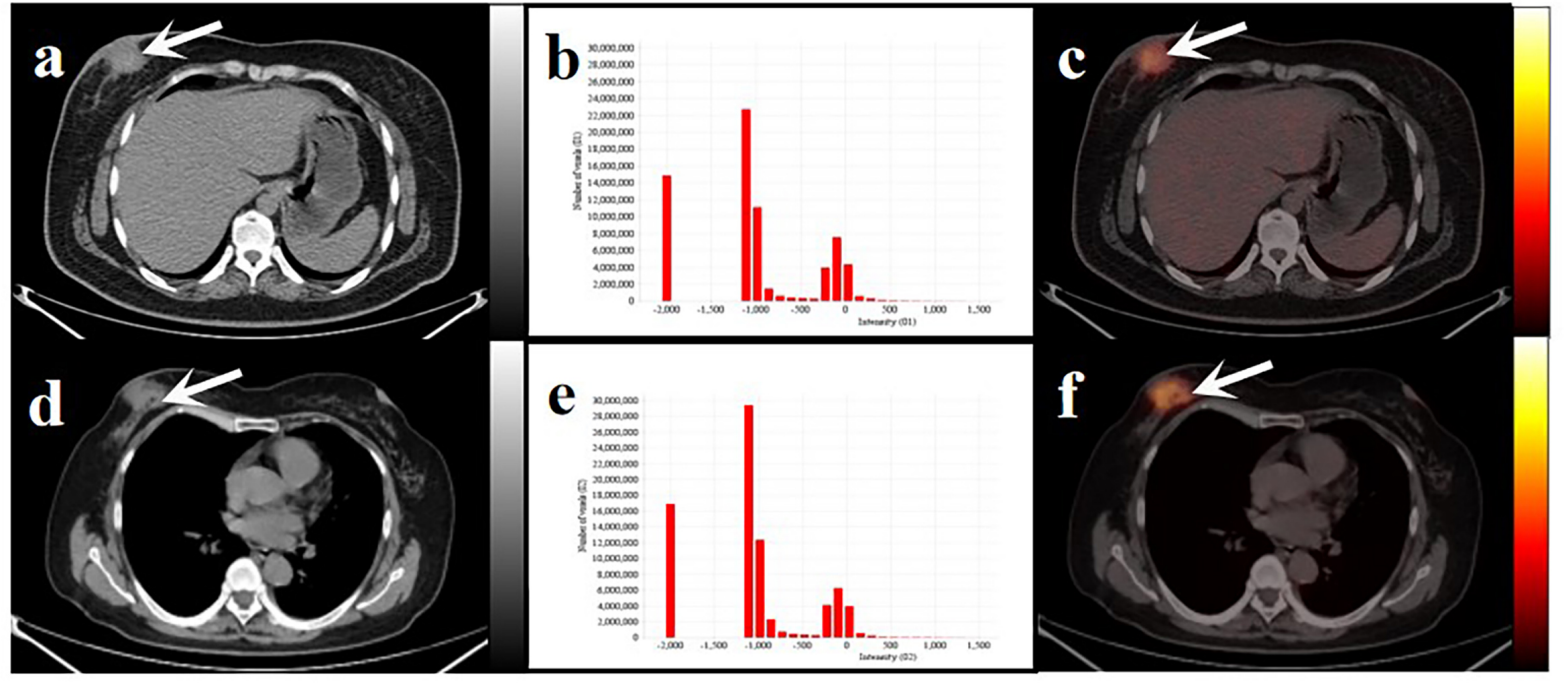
Two women with similar lesions were initially diagnosed with BC. Axial low-dose CT images (A, D) and infusion PET/CT images (C, F). Black and white arrows point to the primary lesions located on the right breast. The histograms of CT intensity for the VOI (B, E). The former (age: 32 years, IDC: grade 2, molecular type: HR+/HER2-, clinical stage: T4N1M0, Rad-score: 0.1647) who underwent neoadjuvant chemotherapy didn’t have any obvious disease progression during the 19-month follow-up. The latter (age:61 years, IDC: grade 2, molecular type: TNBC, clinical stage: T4N3M1, Rad-score: 0.3089) underwent postoperative chemotherapy and died after 20 months. These primary lesions were similar on PET/CT images but showed significant differences in the histograms of the radiomic features and clinical outcomes.
